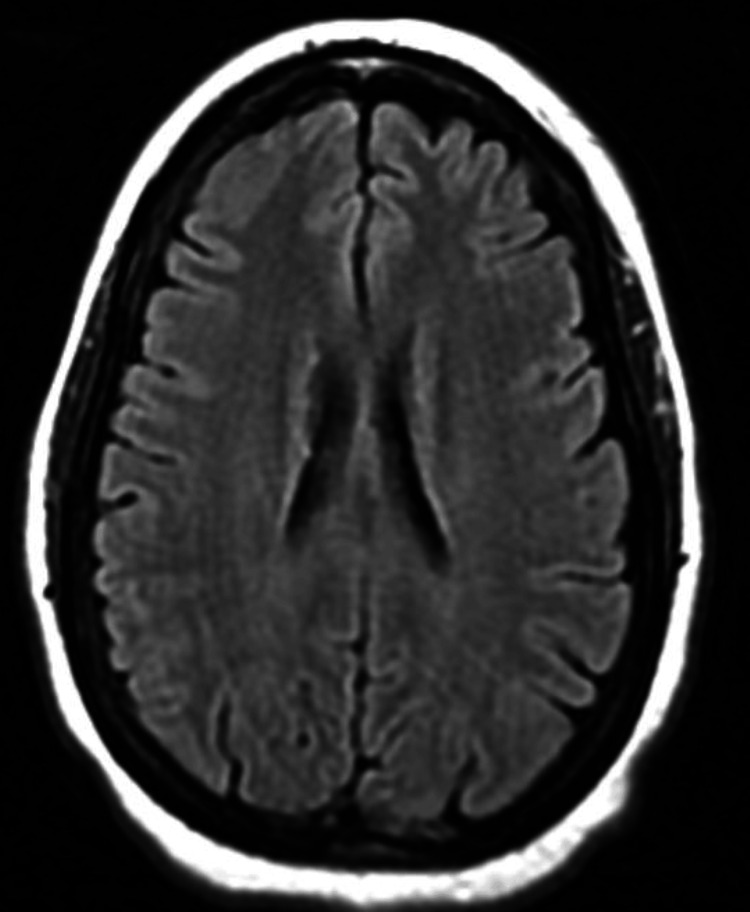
Normal fluid-attenuated inversion recovery sequence of brain magnetic resonance imaging in 2021 (three years prior).
